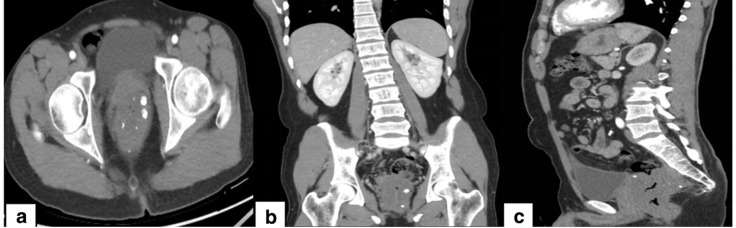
Contrast-enhanced CT of the abdomen and pelvis in 5-mm axial sections (A) with coronal and sagittal reformatted images (B & C, respectively) showing diffuse circumferential thickening of the rectal wall with perirectal serpiginous structures and chunky calcifications likely representing phleboliths. No evident contrast enhancement was seen.
